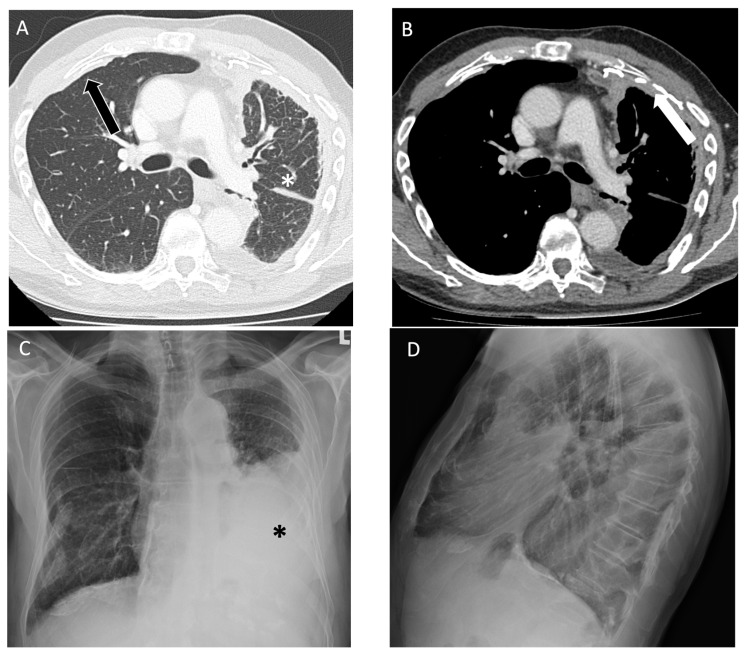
An 89-year-old non-smoking male with asbestos exposure from construction work, diagnosed with epithelioid mesothelioma, later confirmed on video-assisted thoracoscopic surgery biopsy. (A) On axial computed tomography, there is a partly calcified pleural plaque anteriorly on the right (black arrow) and volume loss in the left lung (asterisks) related to a rind of pleural thickening; (B) on the left, the rind of pleural thickening (arrow) has a nodular appearance consistent with mesothelioma and there is a small pleural effusion after thoracentesis that drained 1.2 L of fluid; follow-up (C) posteroanterior and (D) lateral view radiographs one week later demonstrate a reaccumulated moderate-to-large size pleural effusion opacifying the left mid-to-lower lung zone (asterisks).
